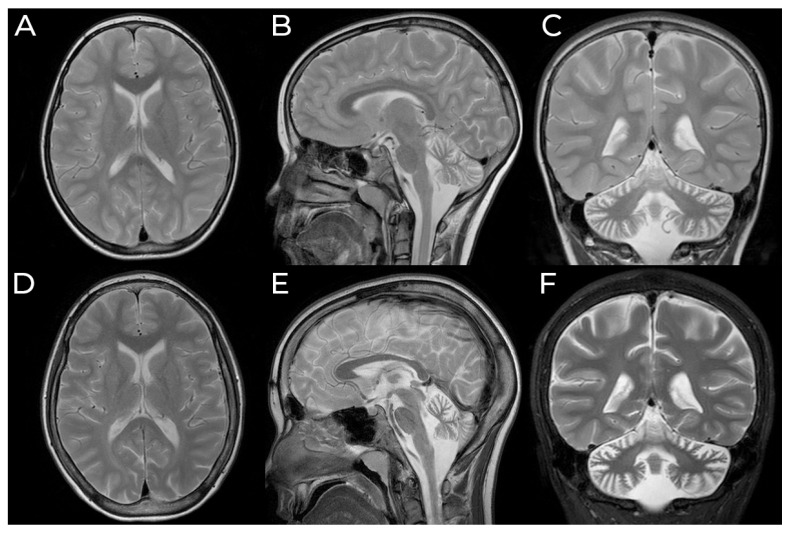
Brain MRI (T2-weighted, axial, sagittal, and coronal planes) of the patient with a mutation c.531C>T in the *CLN8* gene, at the age of 8 years (A–C) and the age of 12 years (D–F) showing bilateral periventricular white matter hyperintensities and progressive cerebellar atrophy.
